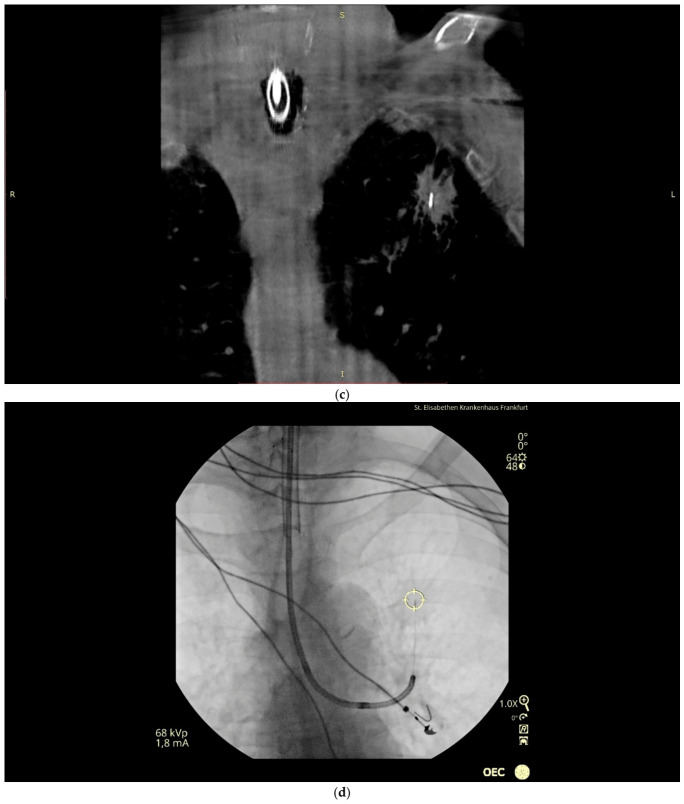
(a–d): Intraoperative CBCT images showing biopsy tool-in-lesion.
